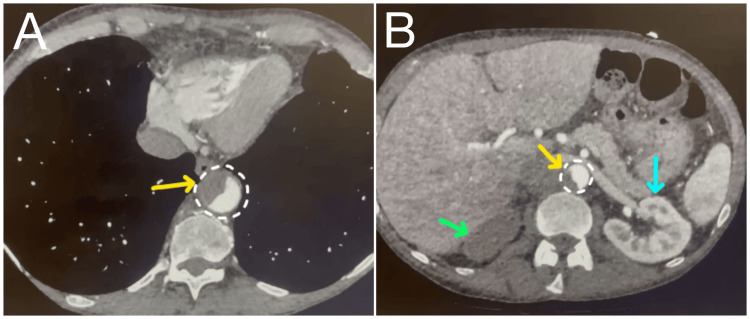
A CT angiogram of the chest (A) and abdomen (B) sections shows a partially occluded and dissected aorta (demarcated by a circle and pinpointed with yellow arrows) from T10-L3 level, confirming type B aortic dissection. The right kidney is infarcted (green arrow), while the left one is normally enhanced (blue arrow).
